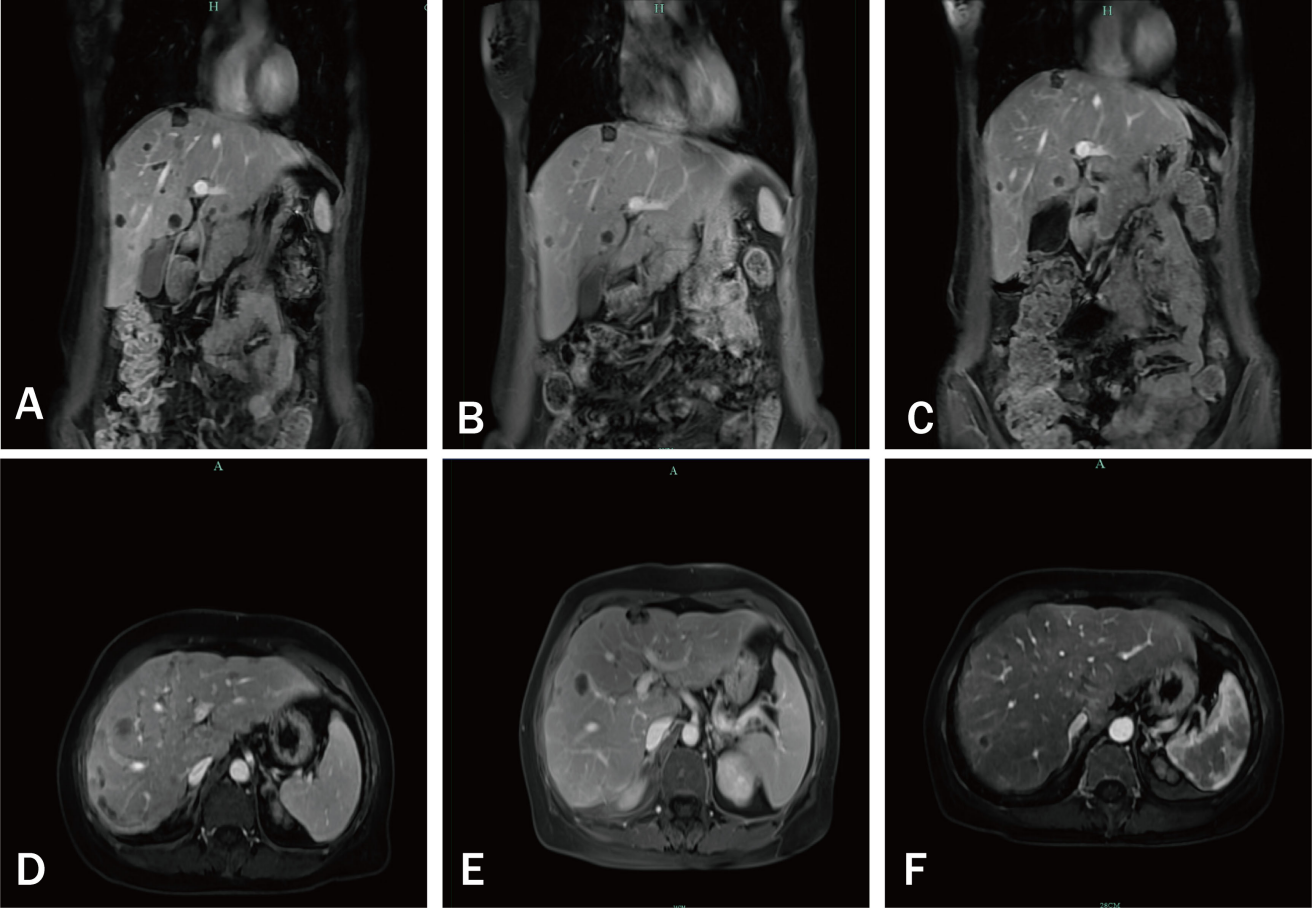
Serial contrast-enhanced T1-weighted magnetic resonance images of the abdomen demonstrating temporal evolution of hepatic lesions. (A, D) 16 September 2024; (B, E) 22 November 2024; (C, F) 6 February 2025.
